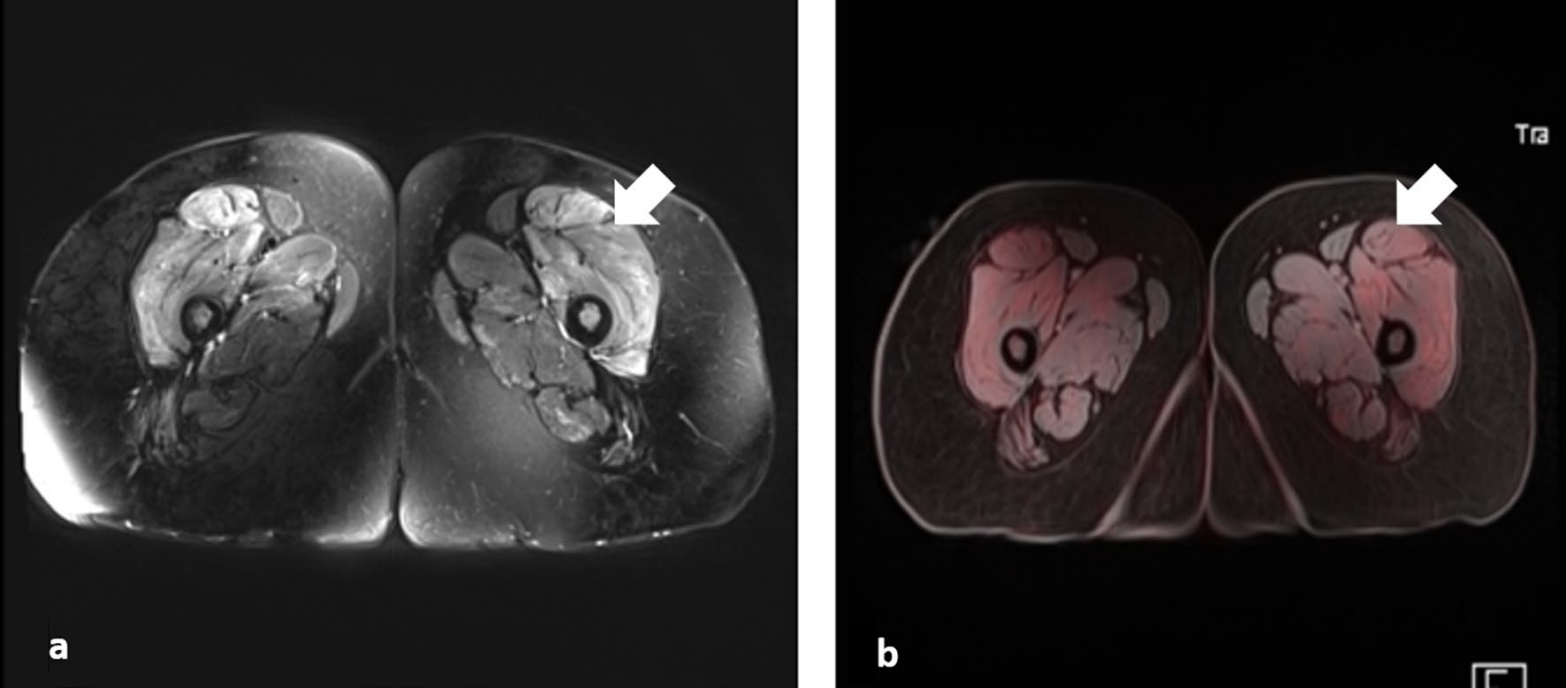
Axial section of thigh muscle in a 33 year old patient with Dermatomyositis showing increased FDG uptake in anterior compartment thigh muscles with corresponding hyperintensity in T2 Fat Saturation sequence.
